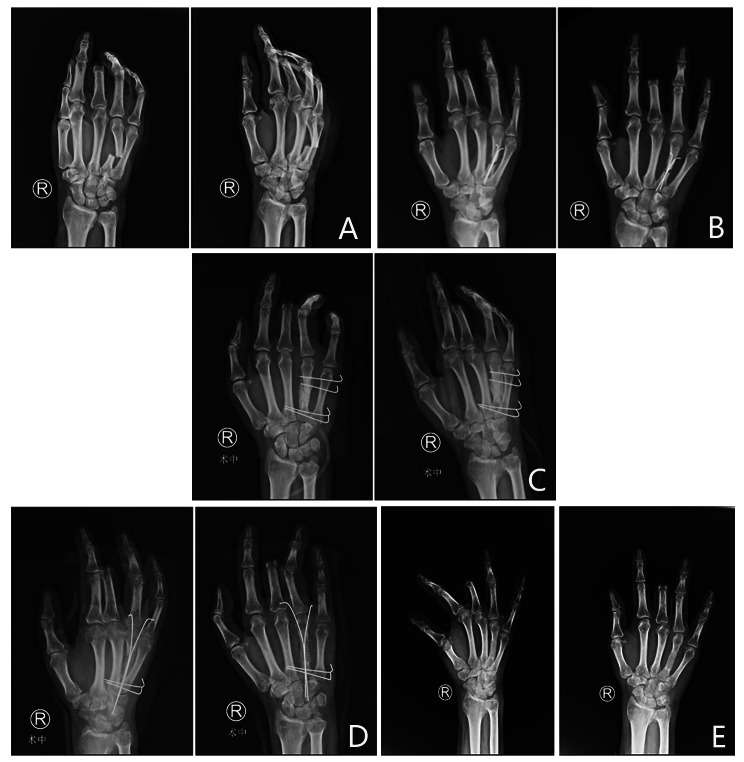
The right hand of a 46-year male patient was crushed by a machine, resulting in open fractures with nerve, vessel, and soft tissue defects. A, Preoperative X-ray. B, X-ray at four months postoperatively, indicating posttraumatic osteomyelitis in his fourth metacarpal. C, After the sinus was removed, the bone defect was filled with antibiotic PMMA cement, and the cement was fabricated into a cylindrical shape similar to the shape of the metacarpal. Four transverse and parallel Kirschner wires were placed for fixation with the adjacent metacarpal. D, At 8 weeks postoperatively, the cement spacer was removed, and the bone graft was carved into a 2 cm⋅ 1 cm ⋅ 1 cm cylindrical shape similar to the shape of the metacarpal. Two crossed Kirschner wires were used to fix the fracture. E, At eight weeks postoperatively, bone graft union was observed without additional infection
